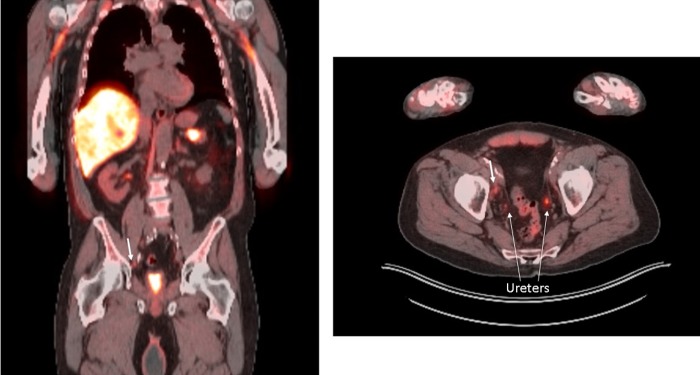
Flucicolvine PET/CT scan showing 1.1 × 0.5 cm mildly enlarged *right* pelvic sidewall lymph node with focally increased uptake suspicious for metastatic prostate cancer. The *thick white arrows* point to site of positive lymph node. *Thin white arrows* point to ureters. PET = positron emission tomography.
